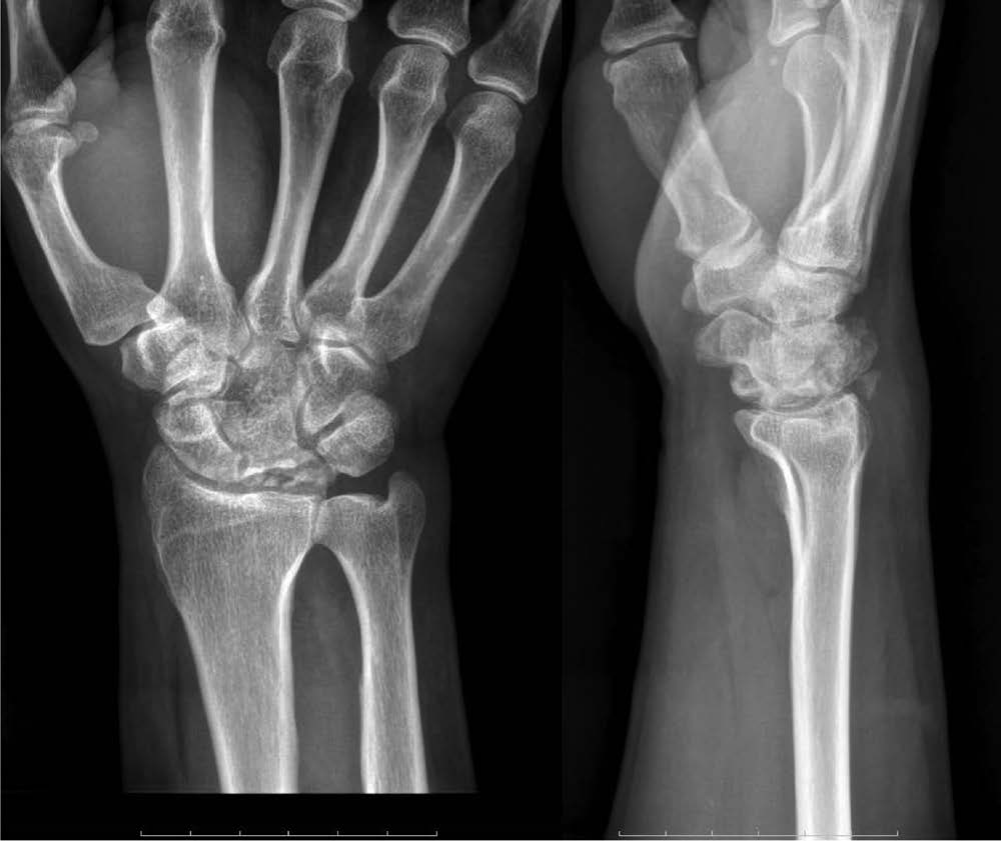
Preoperative radiograph showing abnormal morphology of the right lunate bone, and patchy high-density shadows beside the triangular bone.
